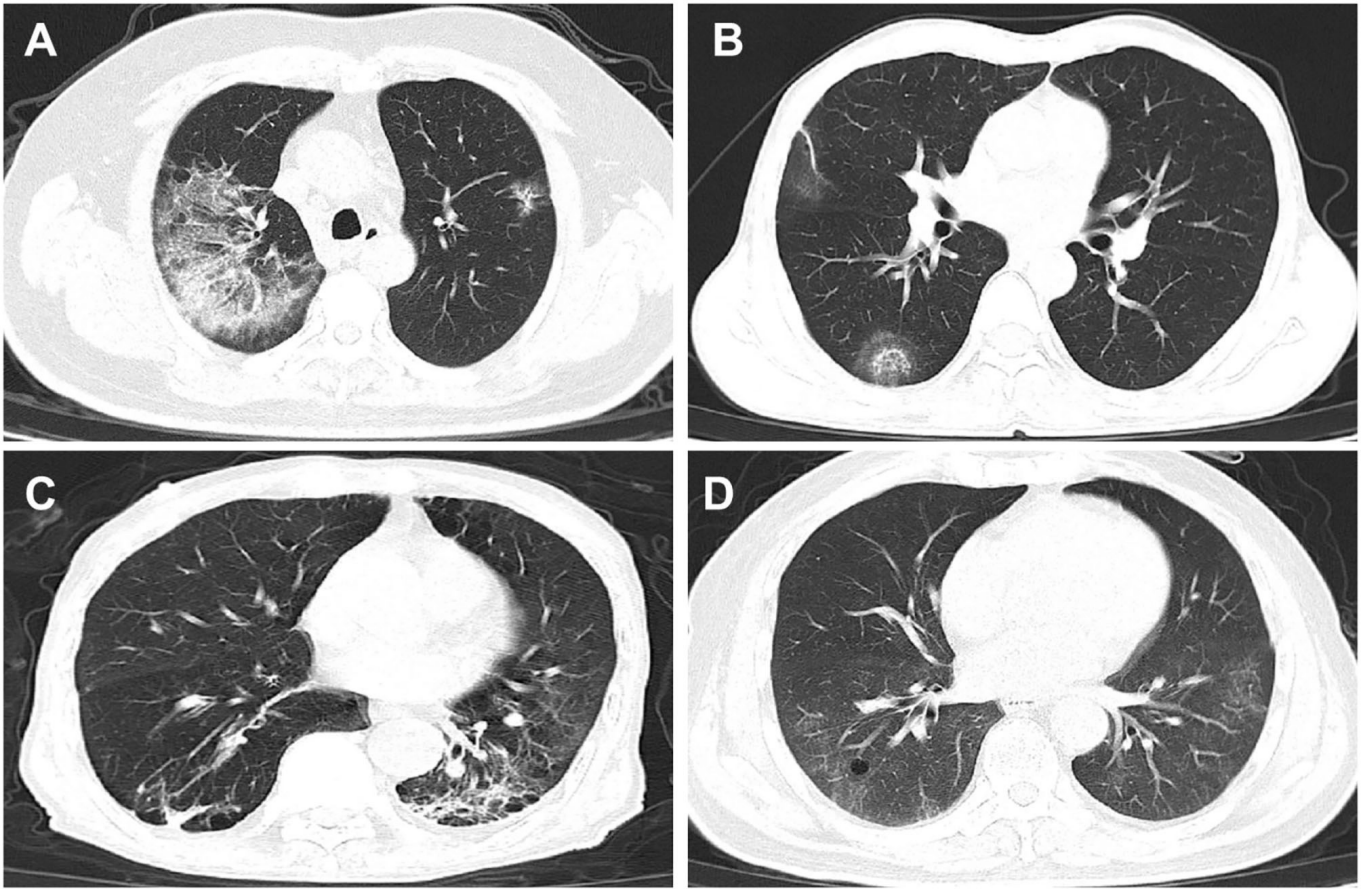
Selected CT graphs of COVID-19 patients infected by the omicron variant of SARS-CoV-2 in Suzhou, China in 2022, taken upon hospital admission, showing representative pathological changes in lungs. (A) From a 64-year-old man with fever and cough symptoms. Axial CT image showed GGOs and consolidation in the right upper lobe, taken on the fifth day from illness onset. (B) From a 53-year-old man having cough and fever. CT image showed rounded opacities in the right lower lobe, taken on the eighth day from illness onset. Lesions were peripherally distributed. (C) From a 61-year-old man with fever. CT image showed linear opacities in the right and left lobes, and lesions were peripherally distributed. Image was taken on the fifth day from illness onset. (D) From a 75-year-old man with cough and fever. Axial CT image showed GGOs and cavitation in the right lobe, and lesion distributions were central and peripheral. Image was taken on the tenth day from illness onset.
